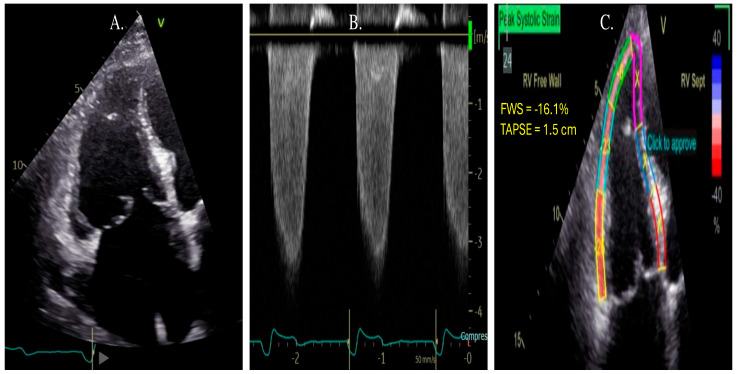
Example of strain analysis in a case of pulmonary hypertension (PH). Panel (A) shows an RV-focused view of a dilated RV with a lack of coaptation of the tricuspid valve but with a visual appearance of normal RV systolic function. Panel (B) demonstrates a continuous wave Doppler signal of severe tricuspid regurgitation, with RVSP of 51 mmHg. Panel (C) demonstrates RV strain analysis with impaired RVLS of −16.1%, which carries incremental prognostic significance in this patient with PH.
